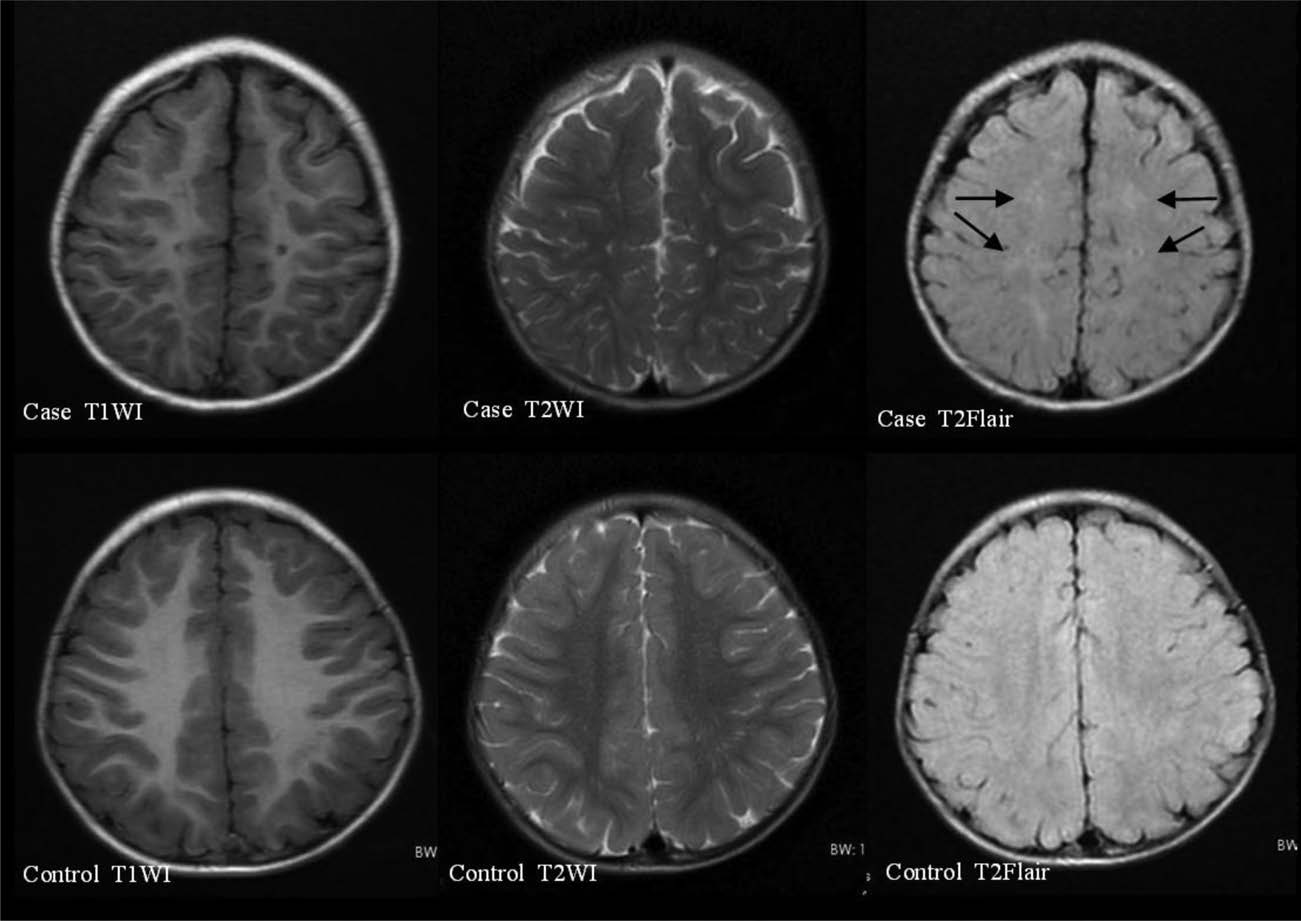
Brain MRI of the patient at 2 years and 3 months showed patchy abnormal signals in the center of bilateral semiovale. MRI = magnetic resonance imaging.
